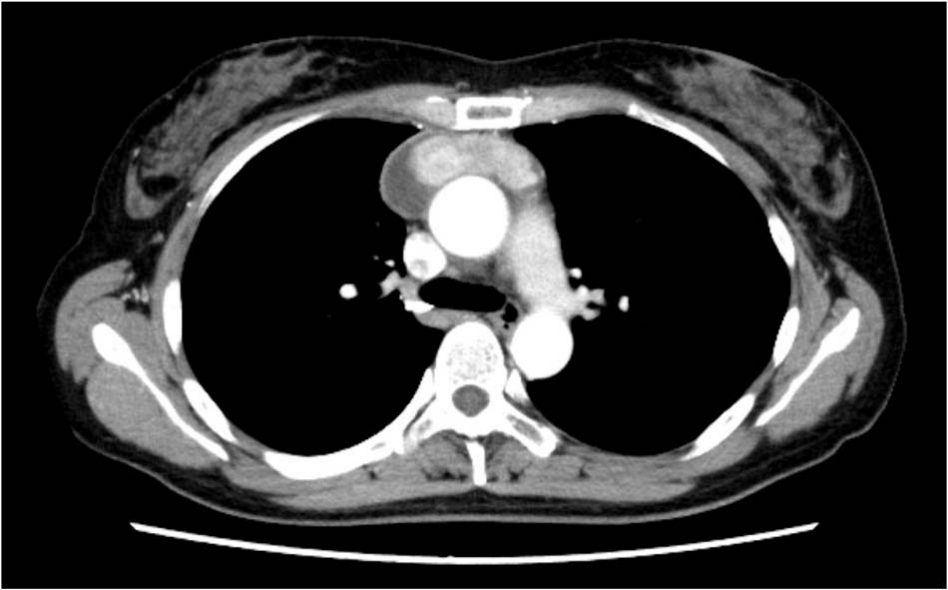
The chest CT scan showing an anterior mediastinal tumor.
